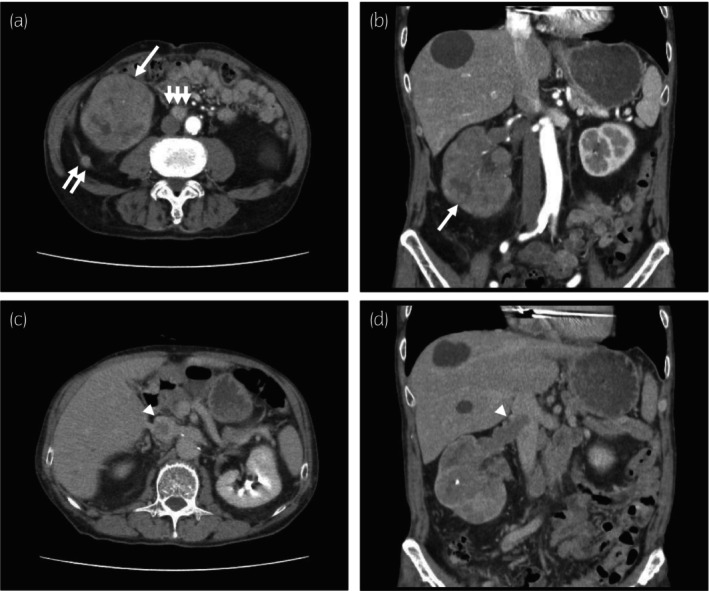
Contrast‐enhanced CT images of the renal tumor, a tumor on Gerota's fascia, lymphadenopathy, and IVCTT. (a, b) Arterial phase images of contrast‐enhanced CT. A renal mass (single arrow), mass on Gerota's fascia (double arrows), and interaortocaval lymphadenopathy (triple arrows) are shown. (c, d) IVCTT images of contrast‐enhanced CT. The tumor thrombus (arrowhead) extends from the renal vein to the IVC beneath the junction of the hepatic vein. (d) shows the cranial end of the tumor thrombus.
